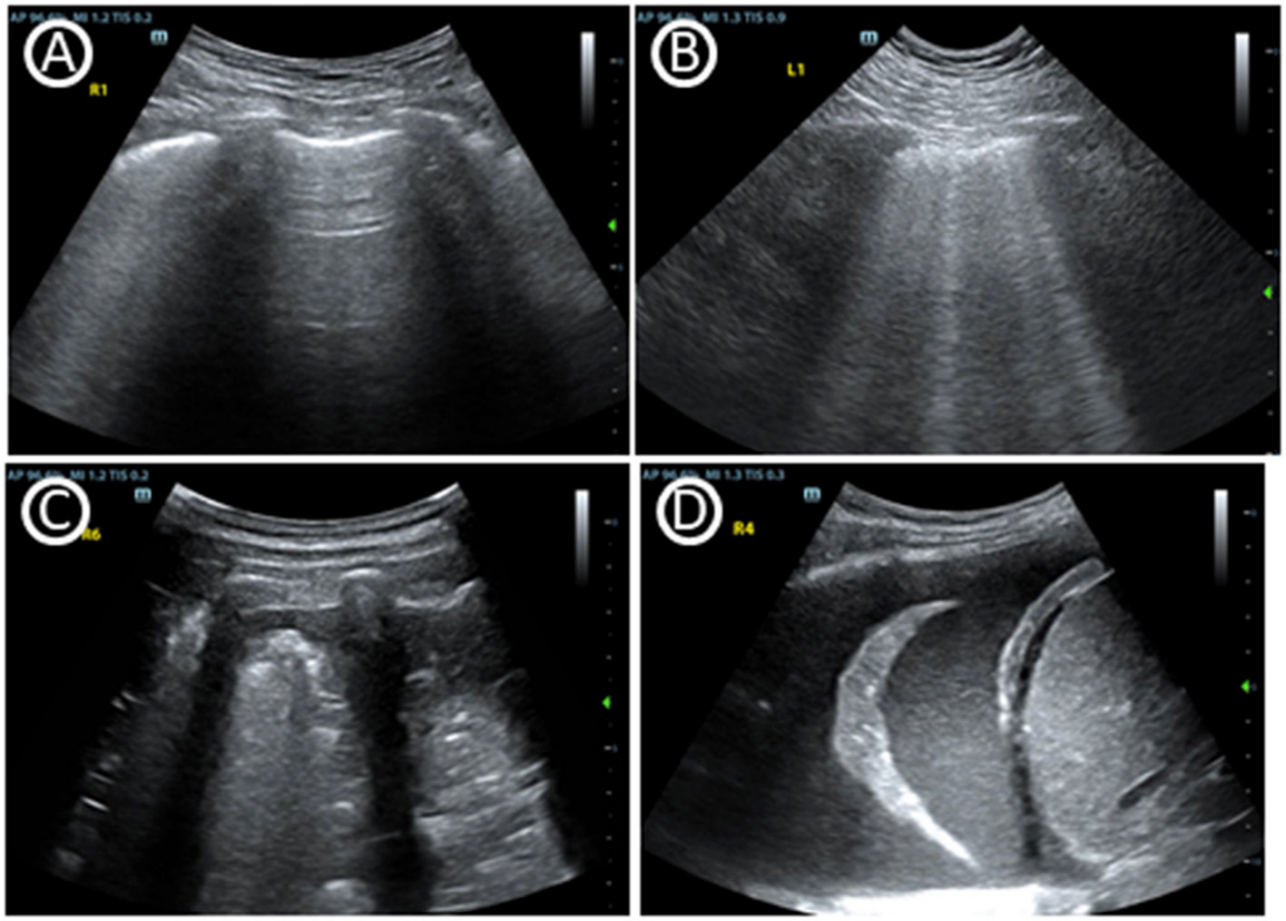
Basic signs of lung ultrasound. (A) A-lines, horizontal reverberation artifacts at a regular distance indicate a high gas–volume ratio below the parietal pleura. (B) Multiple B lines, are vertical artifacts deriving from the pleural line, moving synchronously with lung sliding, usually reaching the bottom of the screen and erasing A-lines. (C) Tissue-like pattern identifies lobar consolidation. (D) Pleural effusion.
